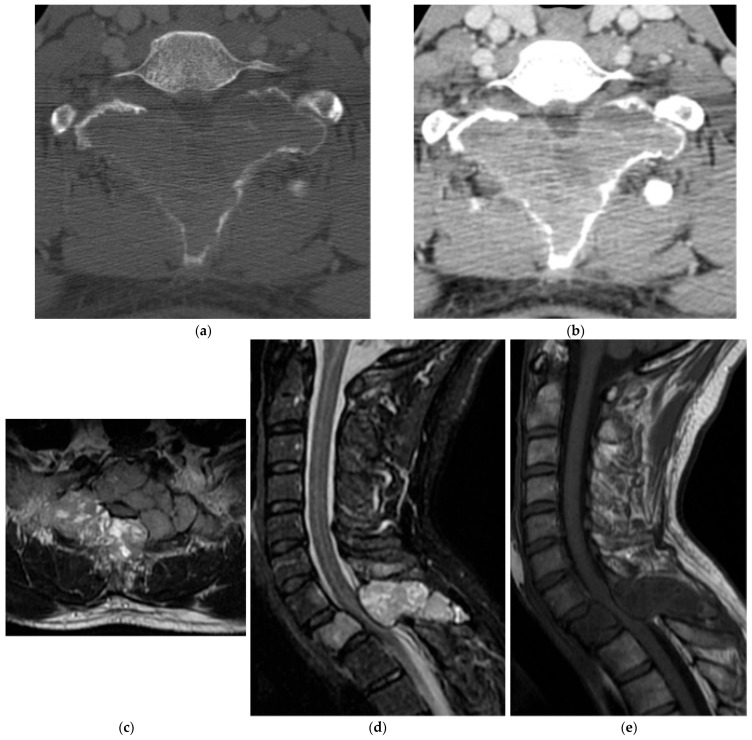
Giant cell tumor. (a) Axial CT bone window and (b) axial CT soft tissue window demonstrate a large, expansile mass in the posterior elements on the cervical spine. There is osseous remodeling with thinning of the cortex. This is a biopsy-proven giant cell tumor. (c) Axial T2 MRI shows an expansile mass with heterogeneously high-signal and low-signal septations in keeping with fibrous components. (d) Sagittal T2 MR shows the same giant cell tumor which demonstrates heterogeneously high signal with involvement of the vertebral body and posterior elements. There is severe spinal canal narrowing with compression of the cord. (e) Sagittal T1 MR sequence: giant cell tumor is typically intermediate to low signal on T1 as in this case.
